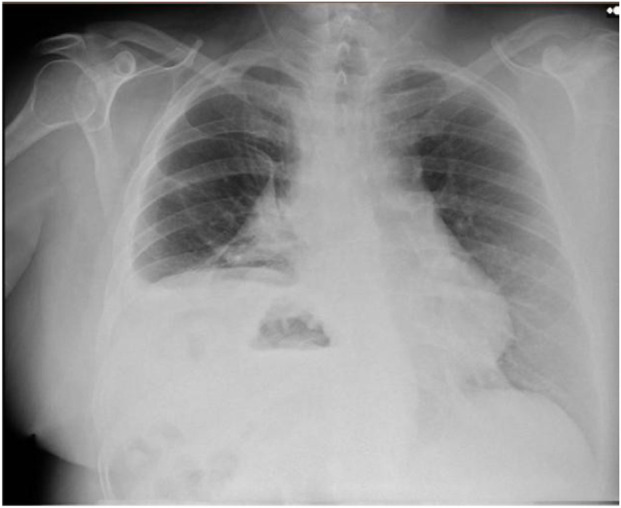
Postoperative Chest X-ray showed no elevation of the left hemidiaphragm.
